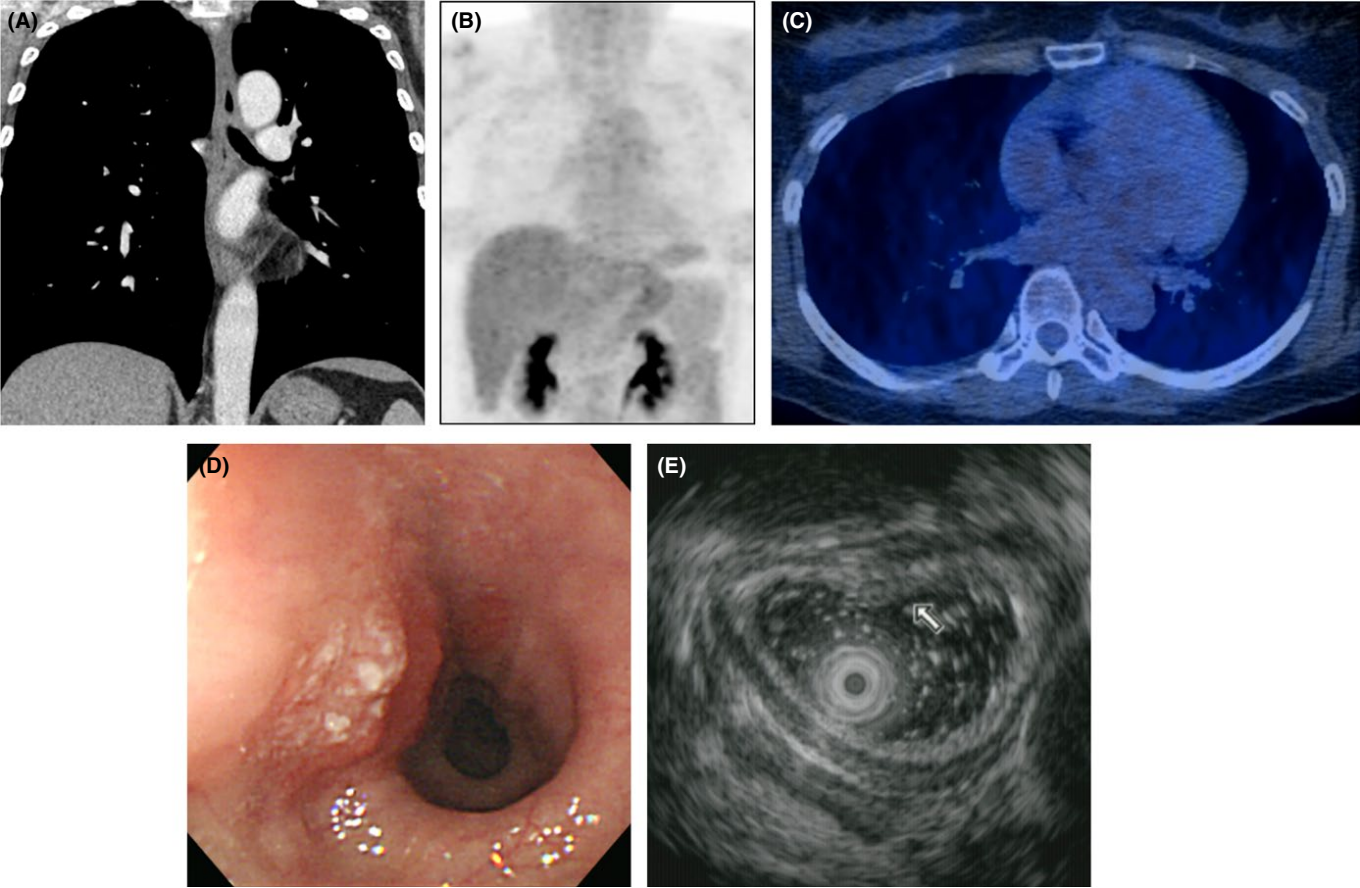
pT1aN0‐stage esophageal squamous cell carcinoma in a 51‐year‐old woman involving the intrathoracic lower thoracic esophagus without lymph node metastasis. (A) Coronal reformatted CT image shows no abnormal wall thickening or mass along the entire esophagus of the esophagus. (B, C) Maximum intensity projection (B) and fused (C) PET/CT image demonstrates no remarkable FDG uptake along the entire course of the esophagus (SUVmax = 2.4 at presumed tumor site). (D) Endoscopy demonstrates a 14‐mm‐sized flat elevated lesion with granular protuberance in the lower esophagus (31 cm from incisor teeth). (E) The lesion is mucosal thickening with preserved submucosal layer (arrow) on EUS. Nodal metastasis was not identified even on pathologic examination
